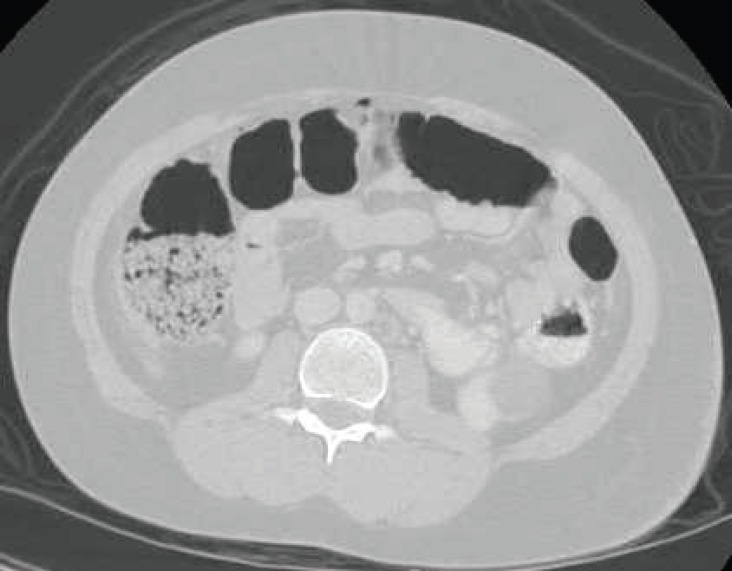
This computed tomographic scan of the abdominopelvic cavity demonstrates small foci of air in the anterior aspect of the abdomen, in favor of traumatic pneumoperitoneum
